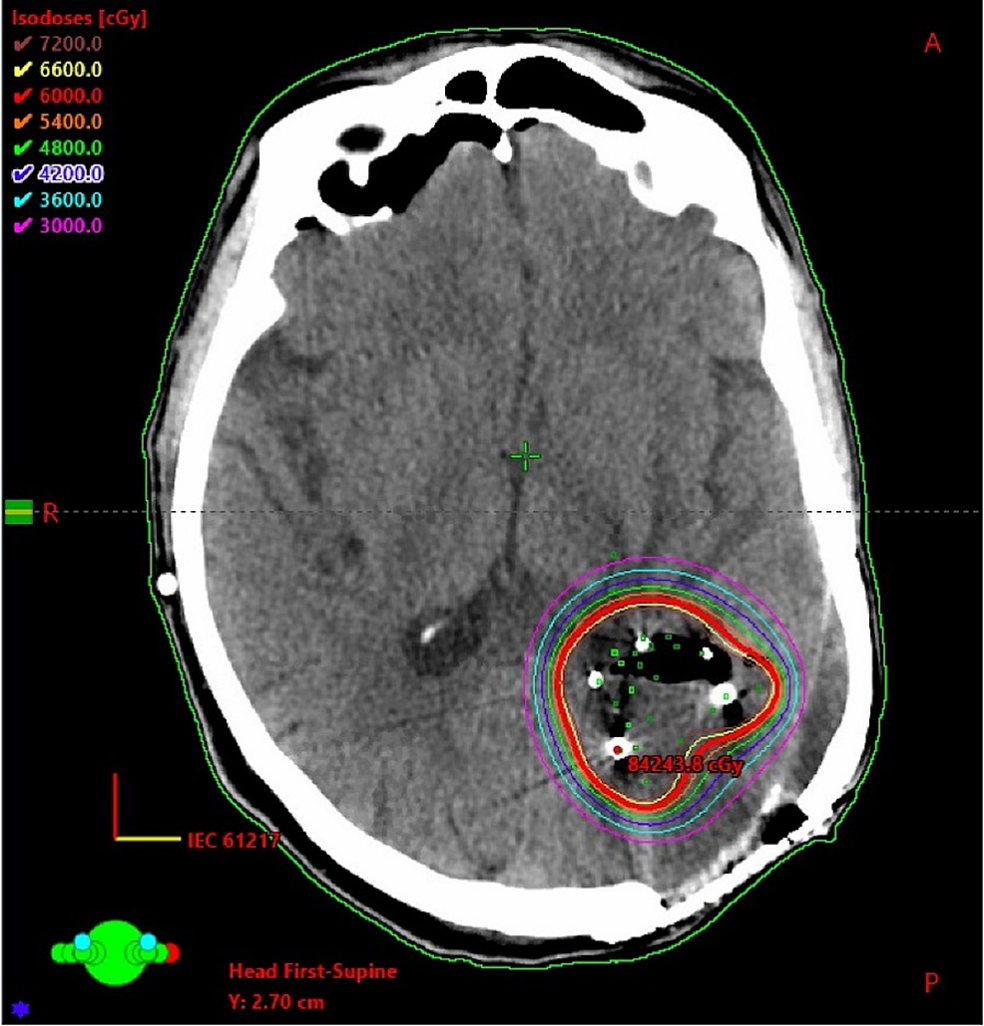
Axial slice of CT Head following Cs-131 GammaTile® placement showing the dose distribution of 60Gy prescribed to a 5mm depth Cs-131: Cesium-131
